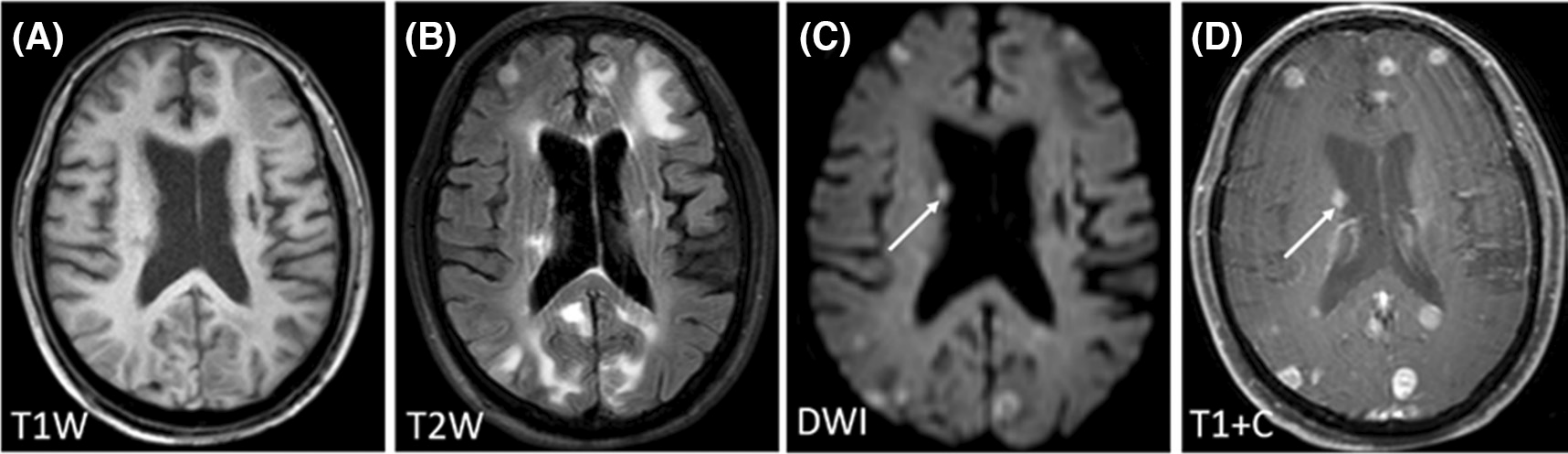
Pattern I of brain metastases in SCLC. Pattern I: A 77‐year‐old woman without history of smoking was diagnosed with BMs 1 year after diagnosis of SCLC. The lesions were numerous and located in supratentorial and infratentorial areas. The lesion (white arrow) was located in the right periventricular, with hypointensity in T1WI (A), hyperintensity in T2WI and DWI (B, C), and homogeneous enhancement (D). BMs, brain metastases; DWI, diffusion‐weighted imaging; SCLC, small cell lung cancer.
